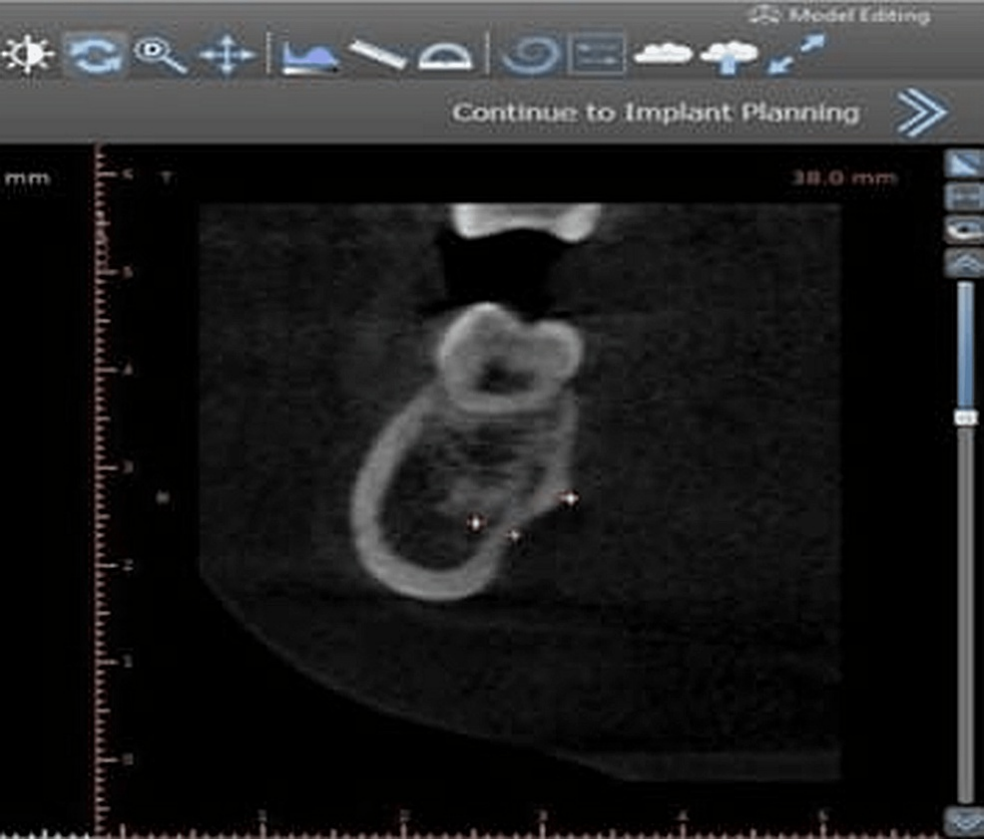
CBCT with three reference point
